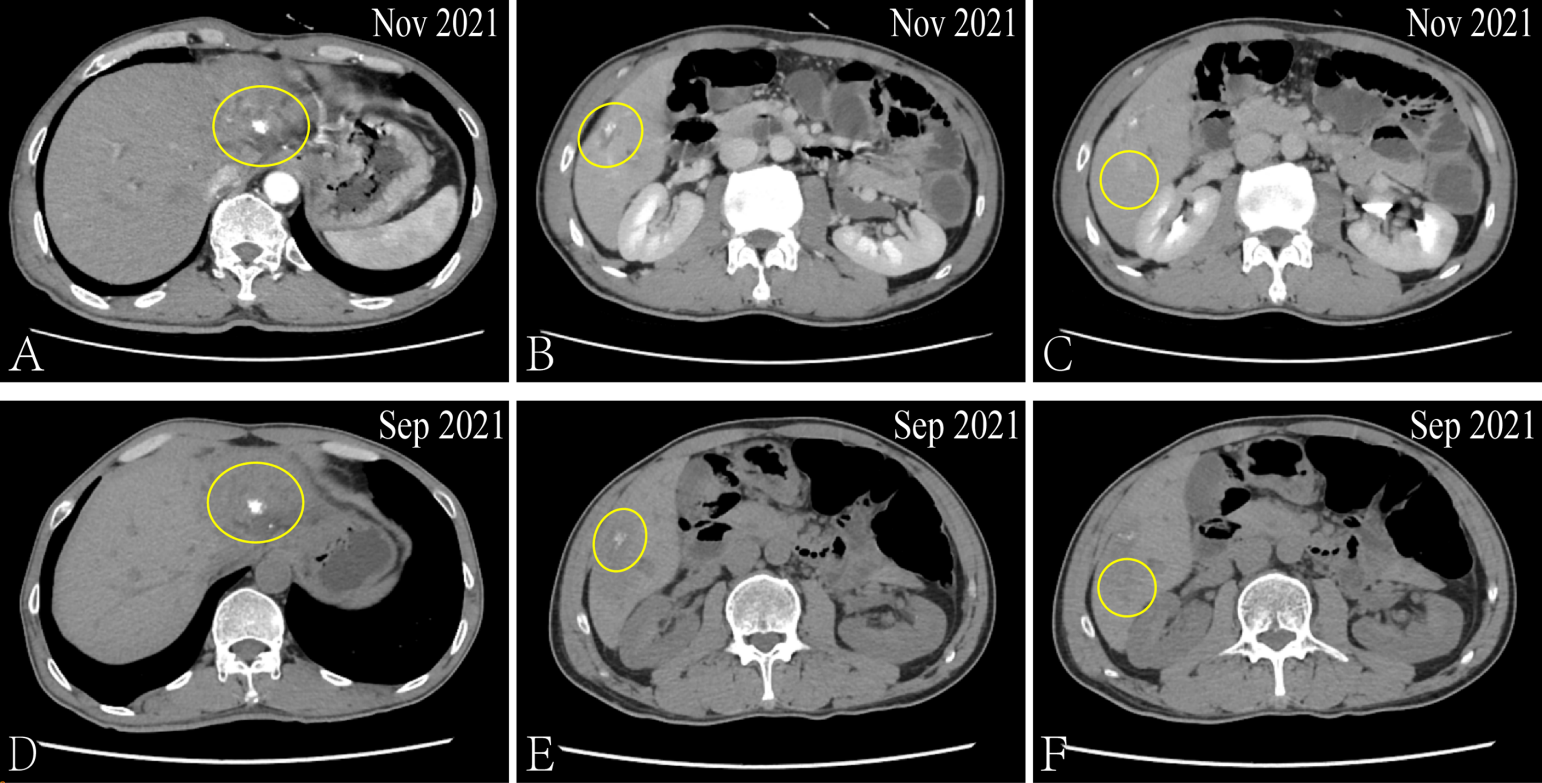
Via* CT to contrast the curative effect between the Nov 2021 and Sep 2021. The size of the metastatic lesion which in the left and caudate lobe of liver is reduction from 3.3 × 2.6cm to 2.8 × 2.2cm (A, D). The size of the metastatic lesion which in liver S5 is reduction from 2.3 × 1.1cm to 2.0 × 0.9cm (B, E). The metastatic lesion in liver S6 has disappeared (C, F).
